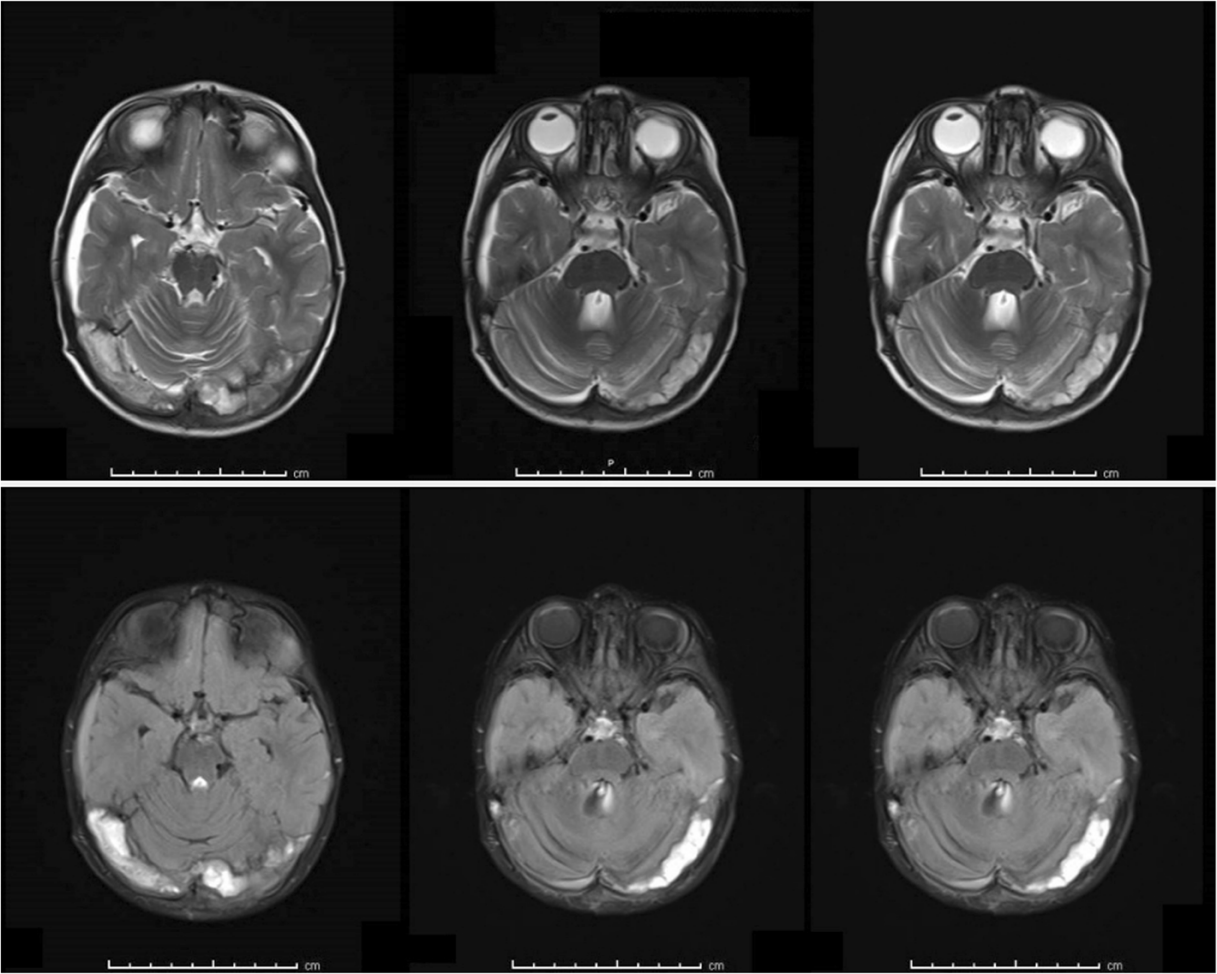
Increased signal intensity on T1, T2 and FLAIR-weighted series compatible with thrombus in right and left transverse sinuses
